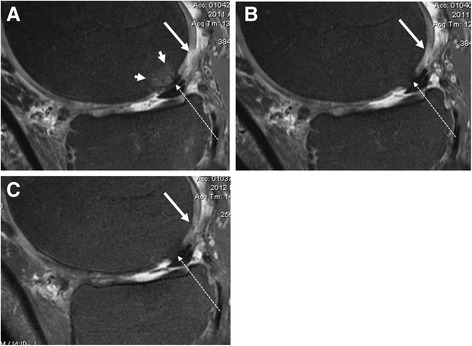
Sagittal intermediate-weighted fat suppressed MRI in treated patient at baseline (a), 3 months (b) and 6 months (c) follow up show improvement of cartilage focal defect of the posterior medial femoral condyle with almost perfect filling at 6 months (long arrows). Also note the grade 1 BML at the central weight-bearing medial femoral condyle disappears at 3 months (a, short arrows). There is a Hoffa-synovitis grade 1 and moderate size tibial osteophytes. There is a femoral intrachondral osteophyte that seems to be slightly increasing in size (dotted arrows)
